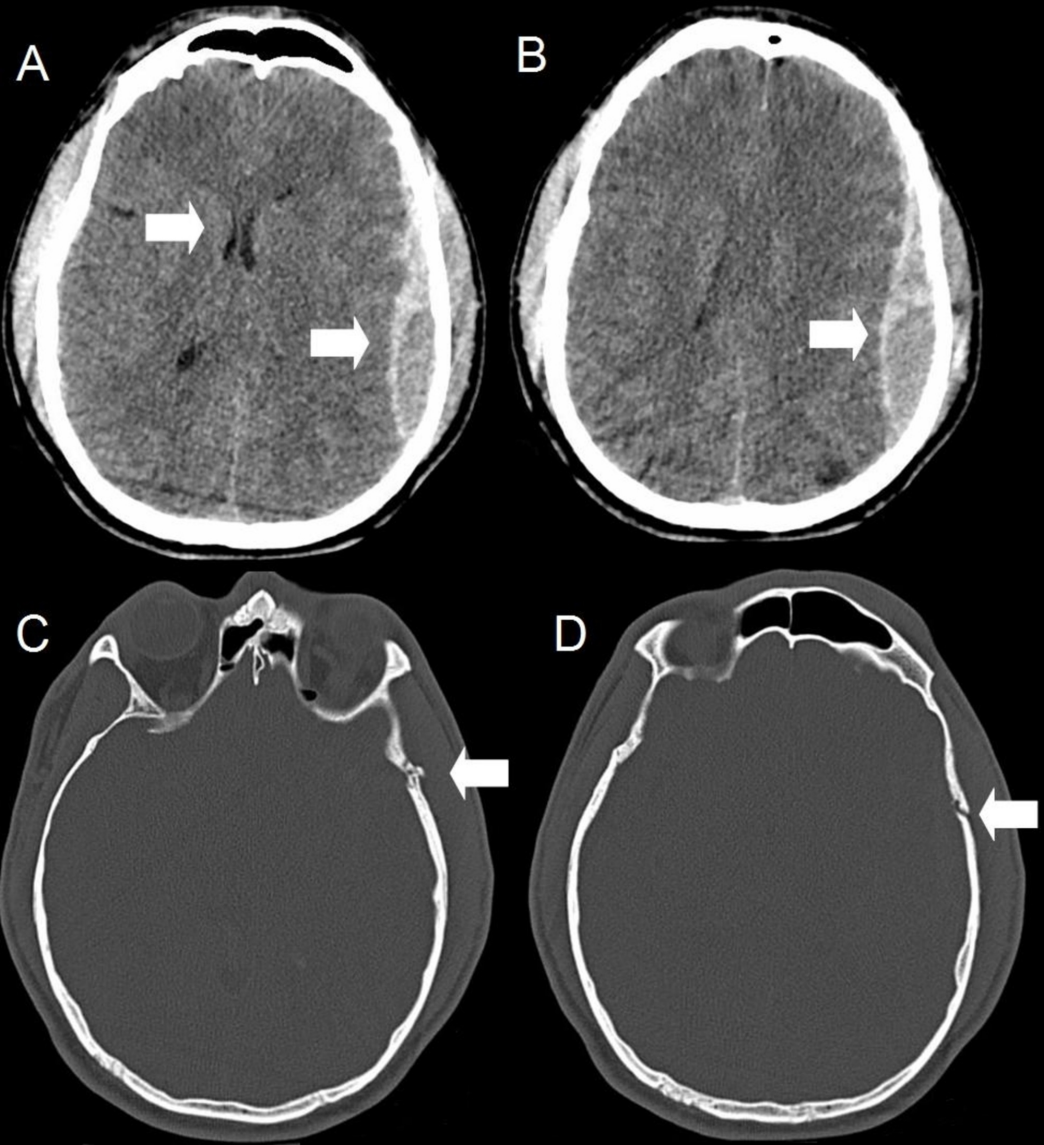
Computed tomography upon initial presentation after blunt trauma Axial (A, B, C, and D) slices show an acute left frontoparietal epidural hematoma of 19-mm thickness with a 5-mm midline shift. There is also a comminuted, non-depressed left temporal bone fracture.
